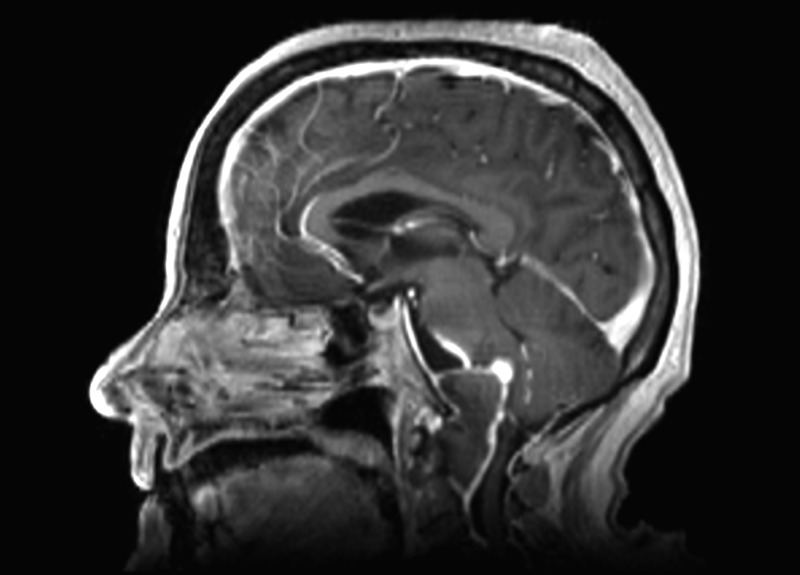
February Sagittal MRI Craniocervical junction mass
